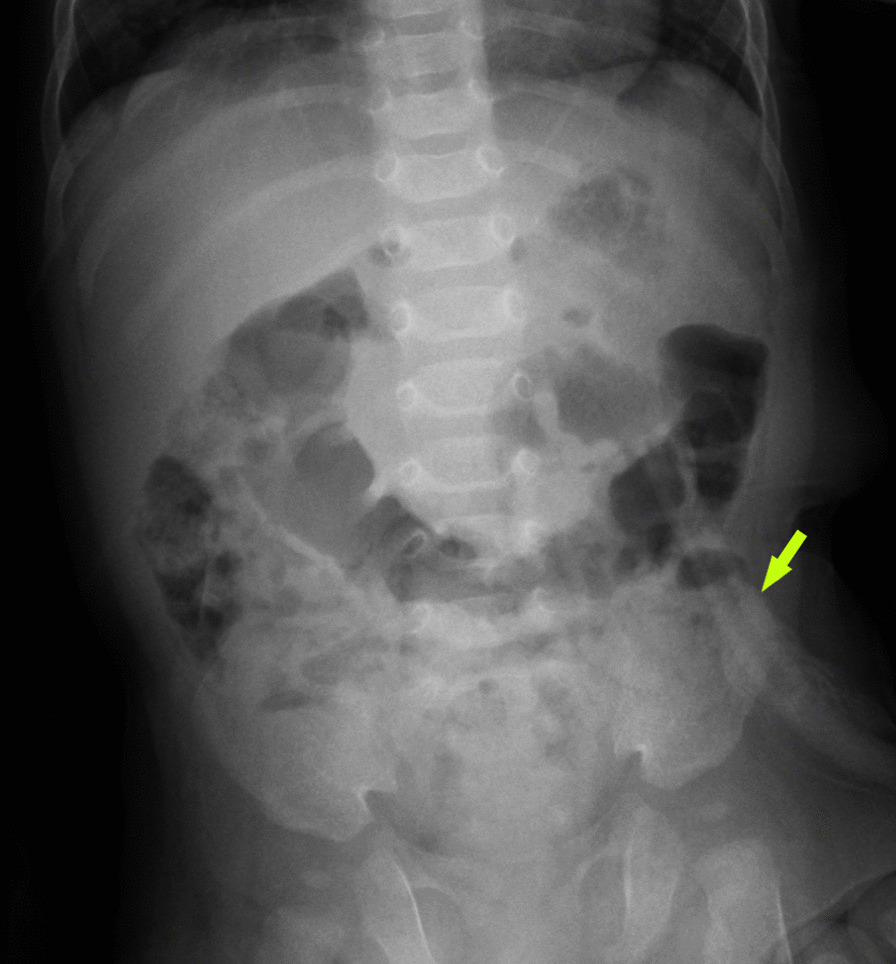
Upright abdominal X-ray of the Pezzer catheter (green arrow) 4 months after placement
